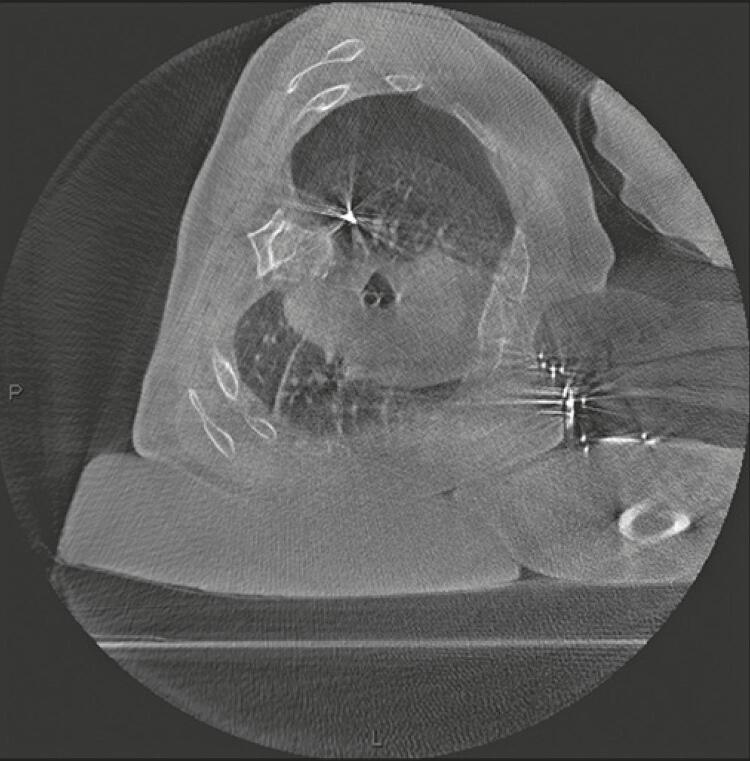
Cone-beam computed tomography demonstrating mild to moderate pneumothorax after microcoil positioning. Pneumothorax had to be drained, once the second lesion localization was impaired by atelectasis
